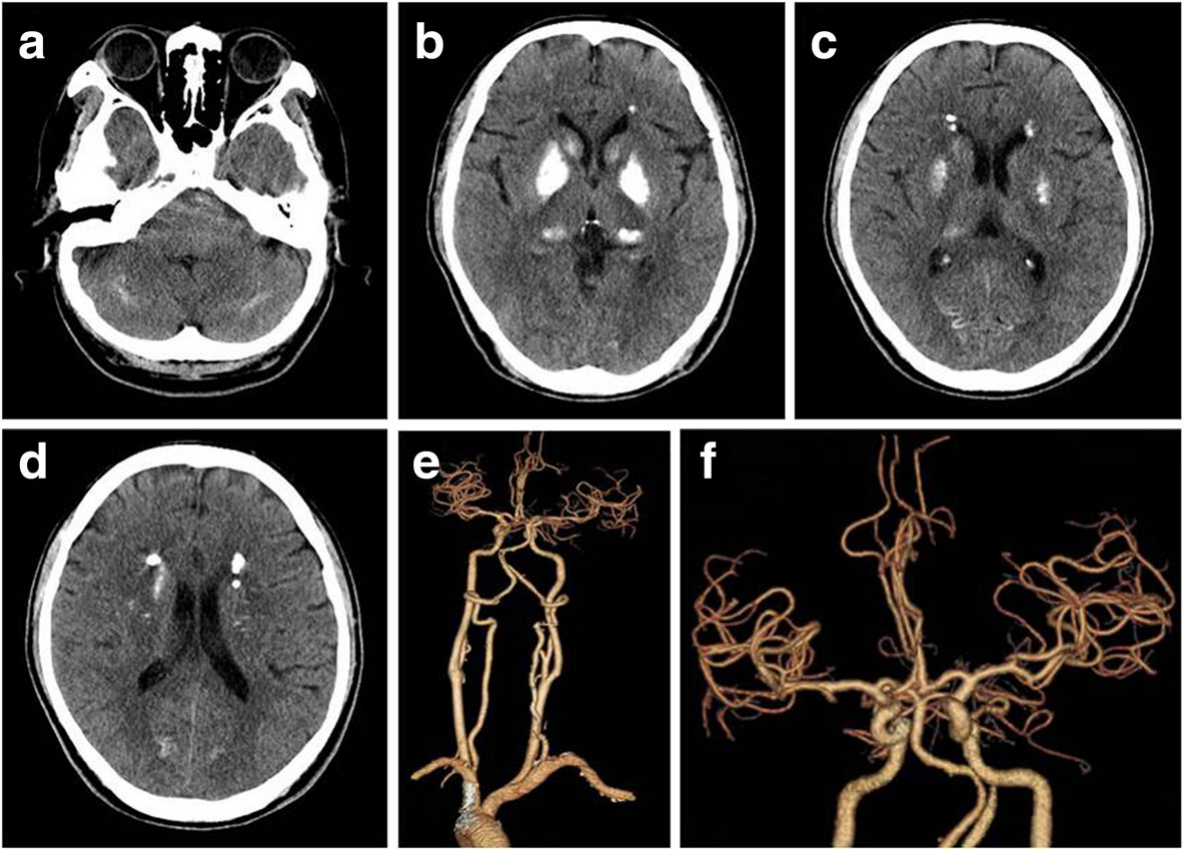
Computed tomography (CT) and CT angiography (CTA). a-d symmetric calcifications in bilateral basal ganglia, caudate nucleus, thalami, subcortical white matter and cerebellum; e-f arteriosclerosis of cervical and cerebral arteries
